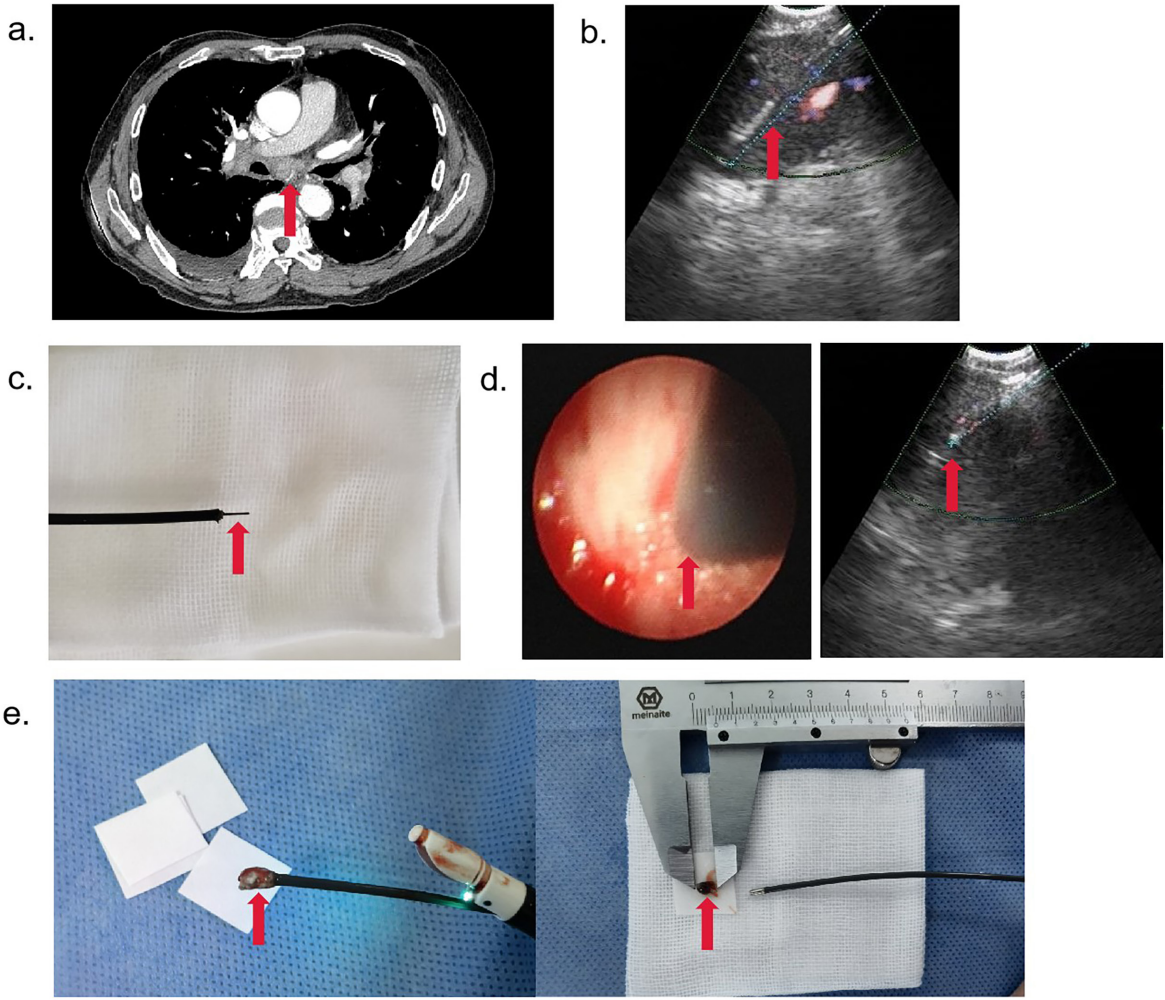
(a) CT image showing the paratracheal mediastinal lesion (Station 7, red arrow). (b) EBUS‐TBNA biopsy (red arrow) of the mediastinal lesion. (c) High‐frequency needle knife. (d) Cryoprobe (ø1.7 mm, red arrow) penetrating the lesion. (e) Sample (red arrow) obtained from transbronchial mediastinal cryobiopsy.
